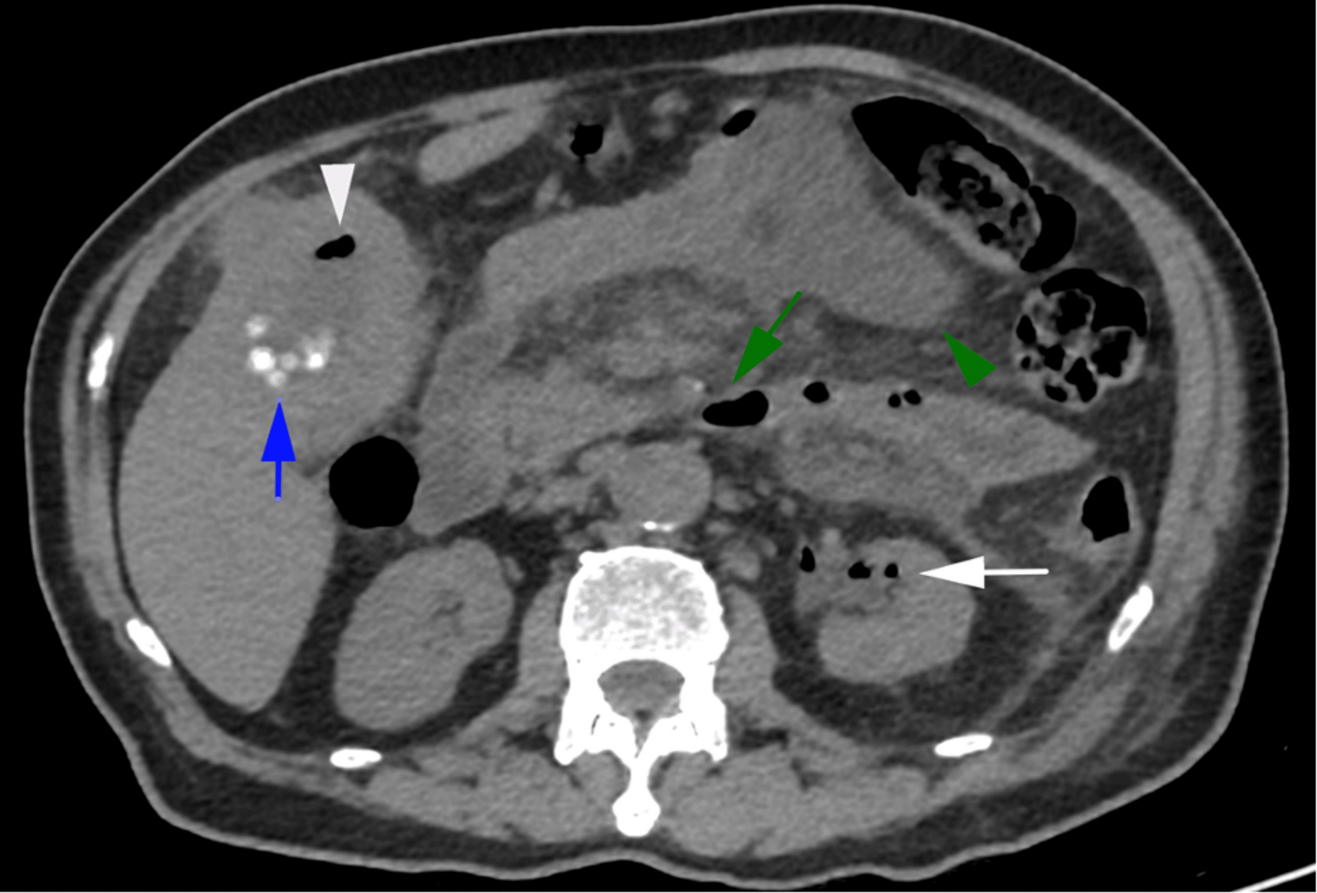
Axial non-contrast CT image of the abdomen demonstrating gas within the left kidney (white arrow), pancreas (green arrow), and gallbladder (white arrowhead) with associated cholelithiasis (blue arrow) and an early, ill-defined peripancreatic collection (green arrowhead).
